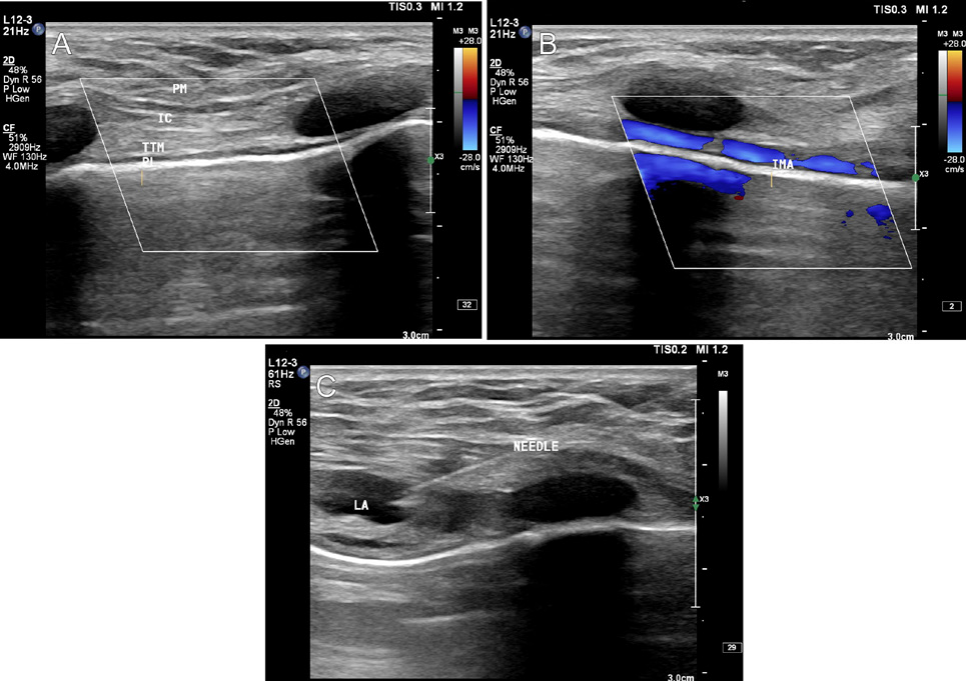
(A) Ultrasonographic depiction of the pectoralis major (PM), internal intercostals (IC), transversus thoracic muscle (TTM), and the pleura (PL). (B) The internal mammary artery (IMA) visualised running between the internal intercostal and transversus thoracic muscle. (C) The in-plane needle tract and tip can be visualised with the local anaesthesia (LA) being deposited in the transversus thoracic muscle plane, pushing the pleura down consequently.
